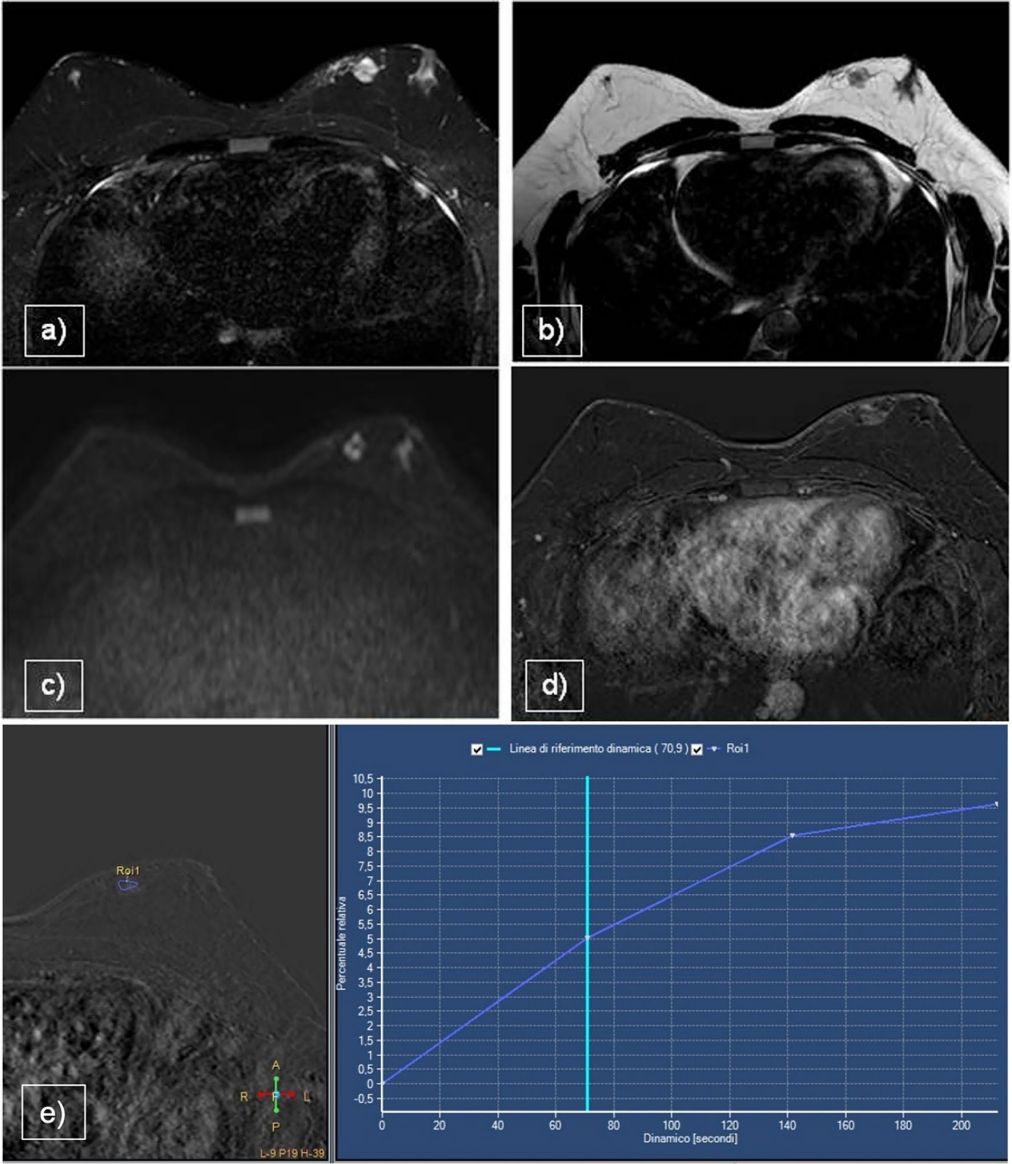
a STIR, b T2WI, c DWI, d CE T1WI, e Time–Intensity Curve. 52-year-old man with a small hard lump appearing between the inner quadrants of the left breast, biopsy was performed with a diagnosis of hemangioma. The vascular tumor demonstrated capsulated margins, DWI restriction and a progressive enhancement (kinetic curve type 1)
